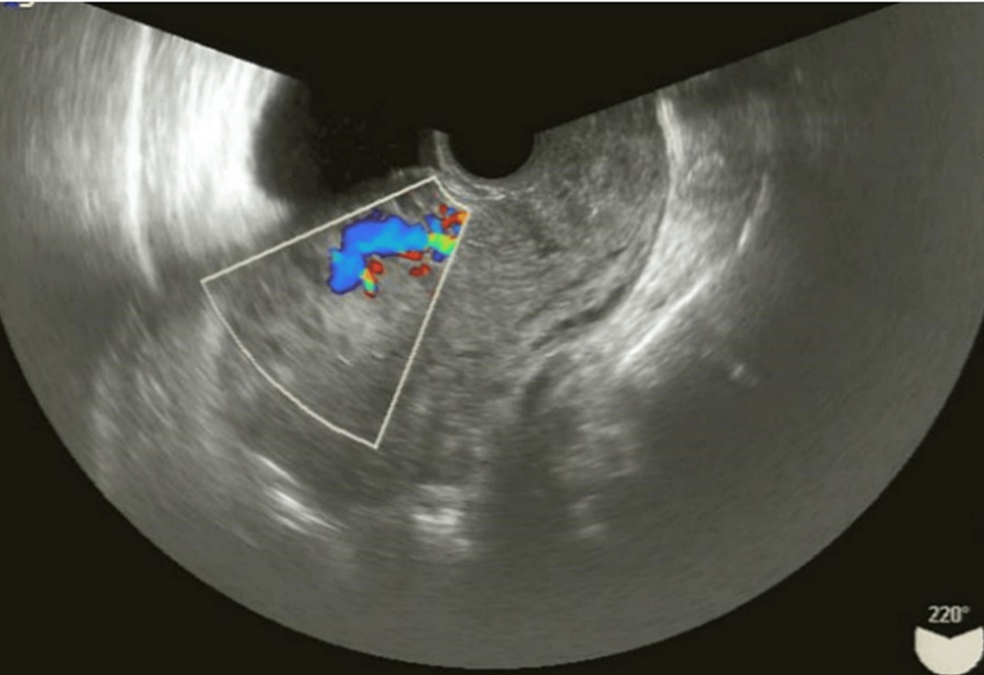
Ultrasound image of placenta accreta Ultrasound scan showing a hyper-echogenic intracavitary lesion measuring 78×36 mm, which appears highly vascular on color Doppler flow examination, consistent with RPOC. RPOC: retained products of conception
